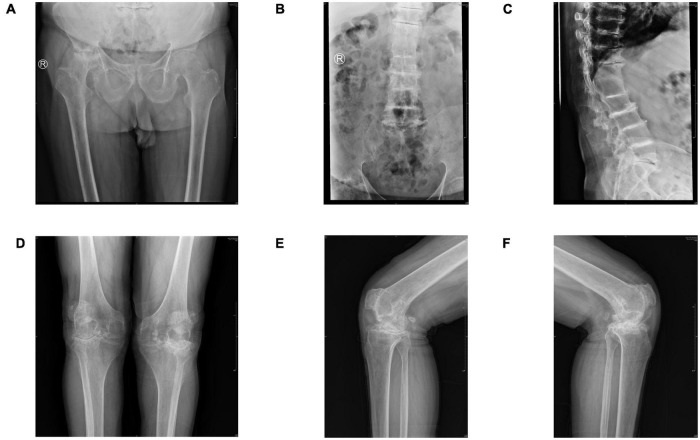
(A) Pelvic radiograph (anteroposterior view) showing an excessive femoral neck-stem angle and fusion of the right femoral head and pelvis. Extensive hyperosteogeny of the right femoral head is observed. (B,C) Lumbar radiographs (anteroposterior and lateral views) showing multilevel narrowing of the thoracic and lumbar intervertebral spaces and lumbar spondylolisthesis, with osteophytosis, calcification, and subchondral osteosclerosis. (D) Radiograph of the knee (anteroposterior view) showing severe degeneration, extensive osteophytosis, significant joint space narrowing, and subcortical osteosclerosis. (E,F) Preoperative radiographs of the bilateral knees (lateral views) showing narrowed joint spaces, loose bodies, severe subchondral osteosclerosis, and extensive osteophytosis.
